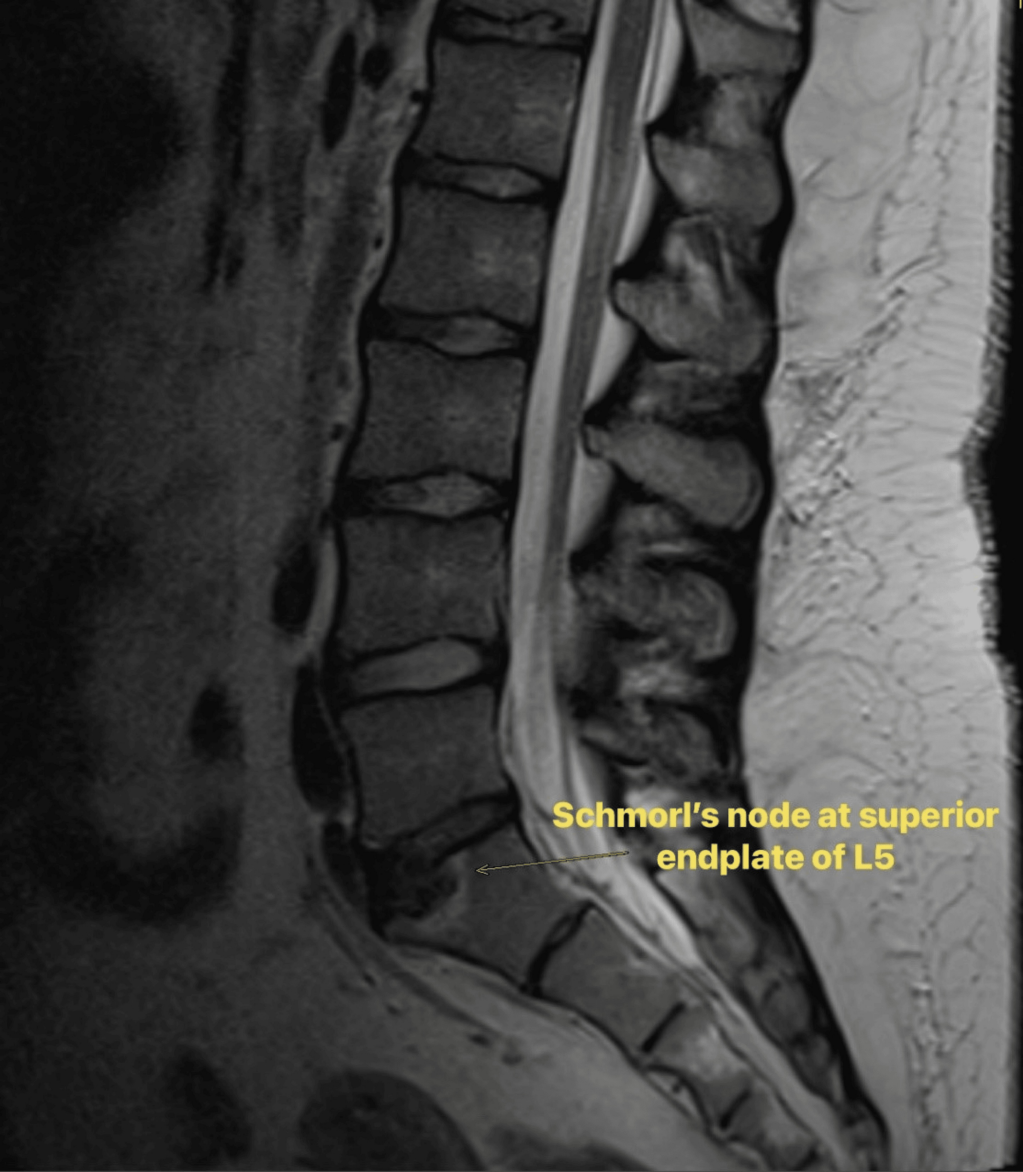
MRI spine showing Schmorl’s node at the superior endplate of L5
